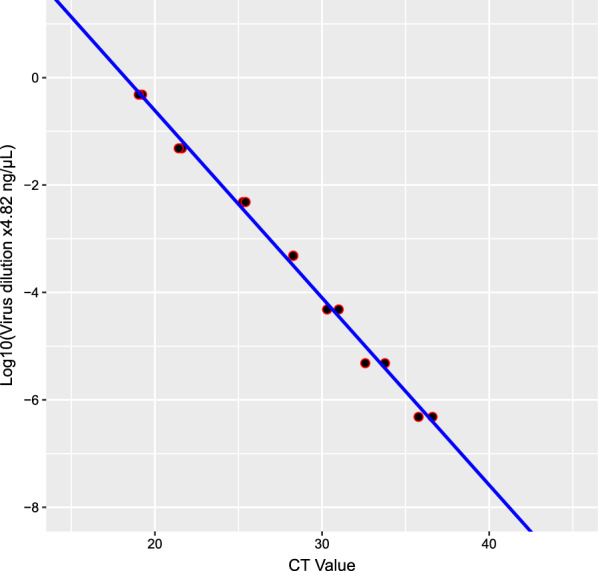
Virus dilution curve and its relationship with CT (cycle threshold)
